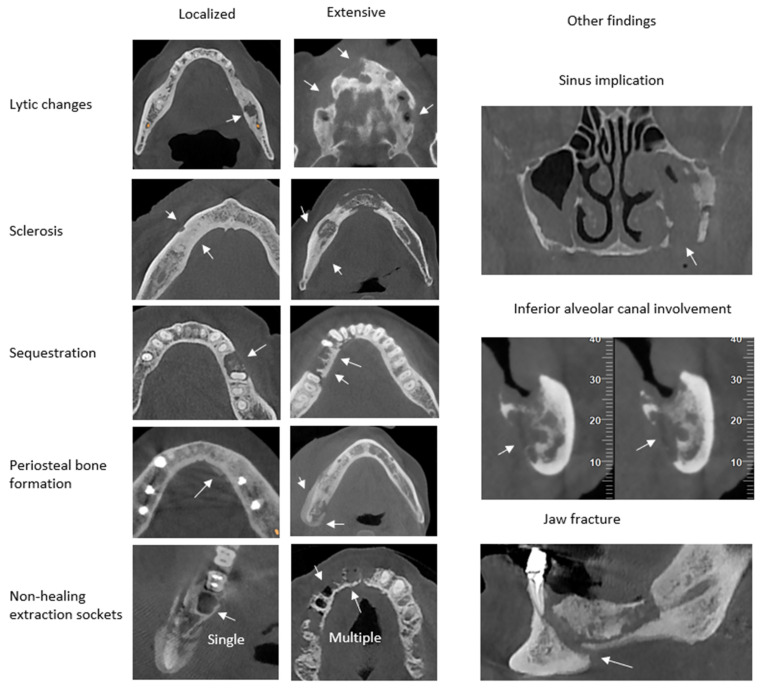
Examples of CRIm scoring for severity of radiologic findings in CBCT images.
